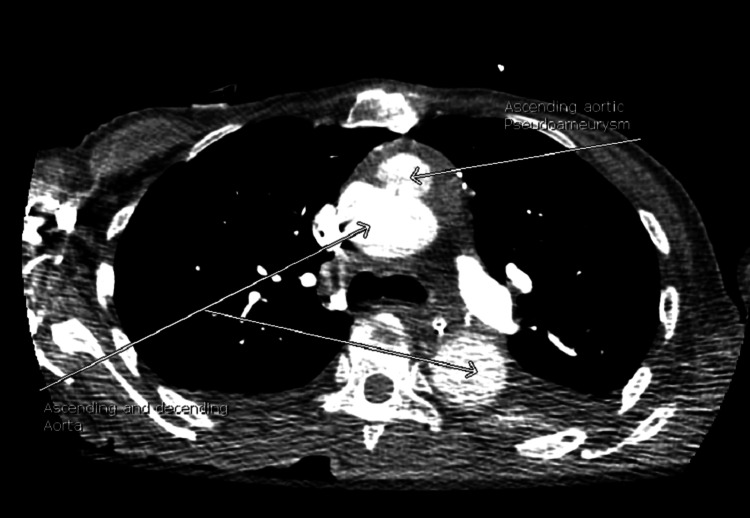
CT angiogram chest depicts ascending aortic pseudoaneurysm.
